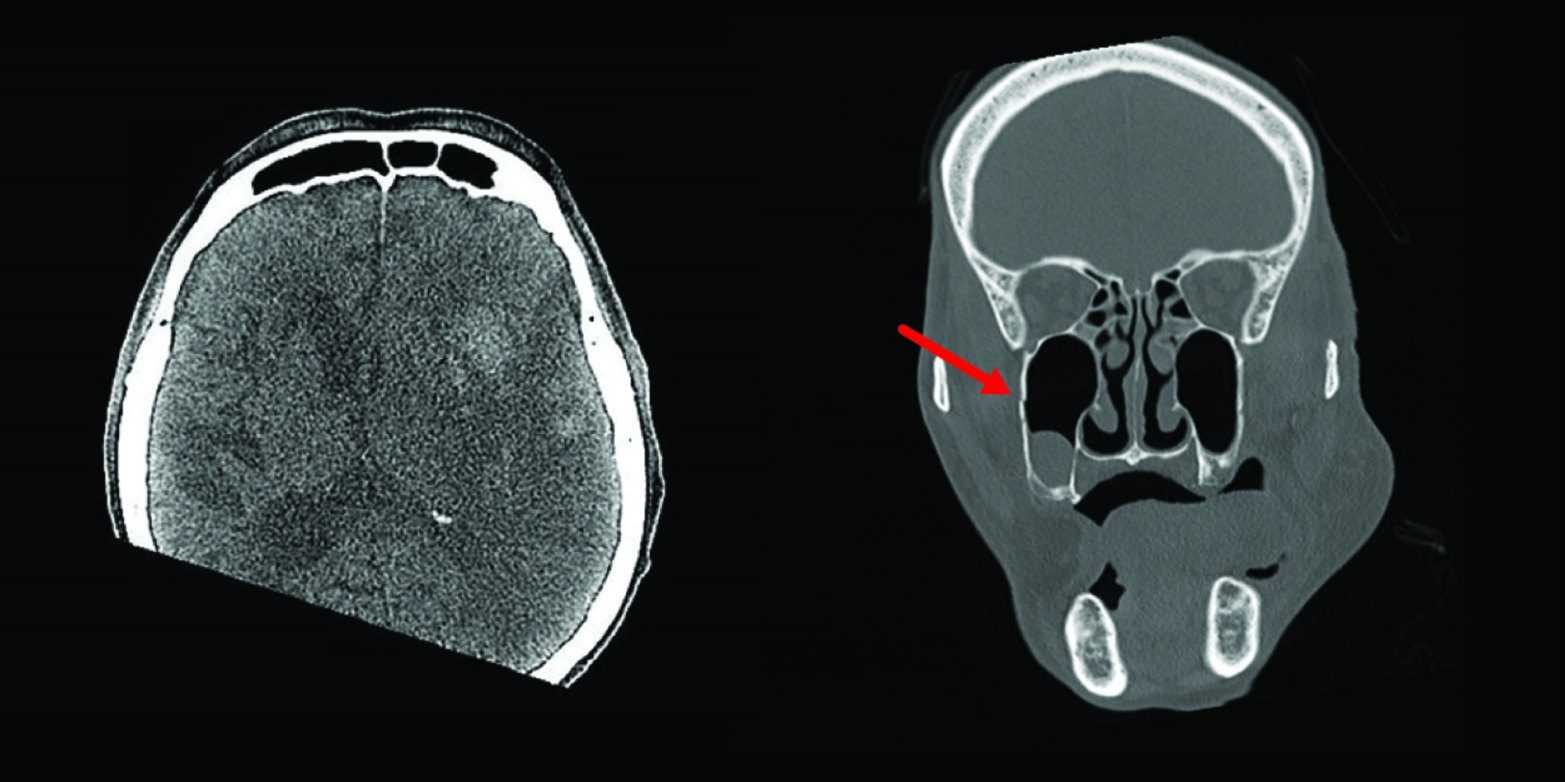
CT Facial Bone Computed tomography (CT) facial bone showing a left middle cerebral artery territory infarct with hemorrhagic conversion and midline shift (left) and fracture of the lateral wall of maxillary sinus (right)
